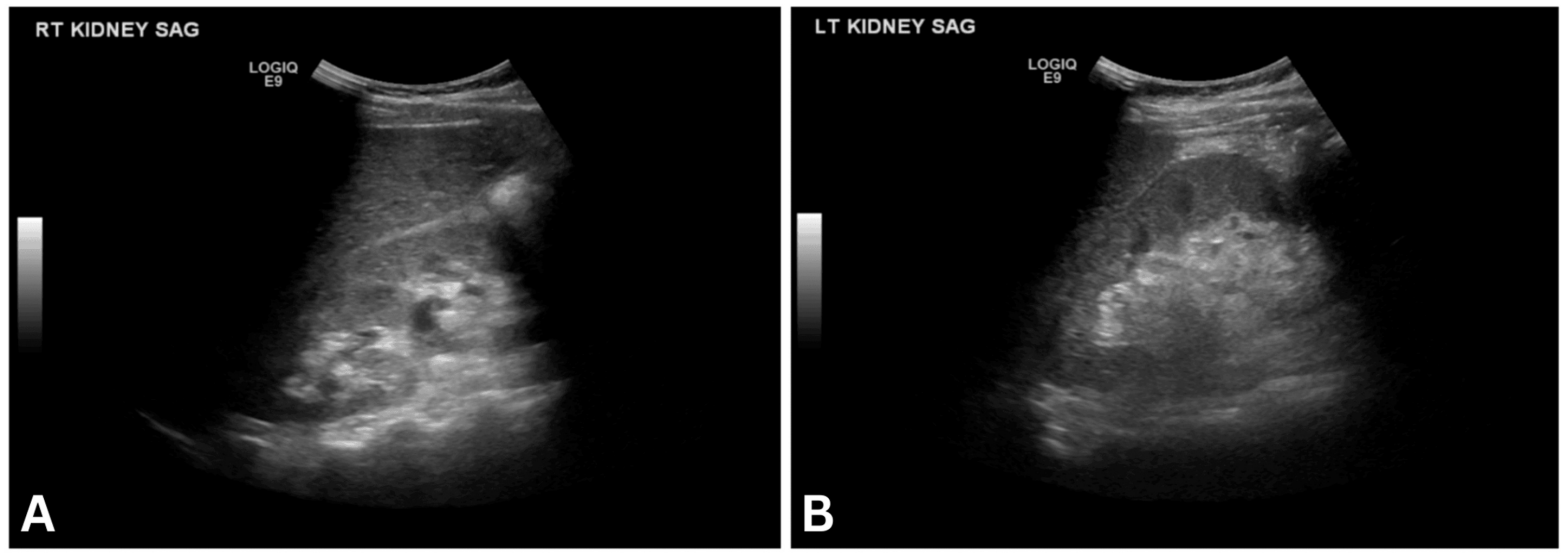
Renal ultrasound image of the right and left kidneys (A) A renal ultrasound image of the right kidney showing increased echogenicity indicative of medical renal disease with no hydronephrosis. (B) A renal ultrasound image of the left kidney showing increased echogenicity indicative of medical renal disease with no hydronephrosis.
